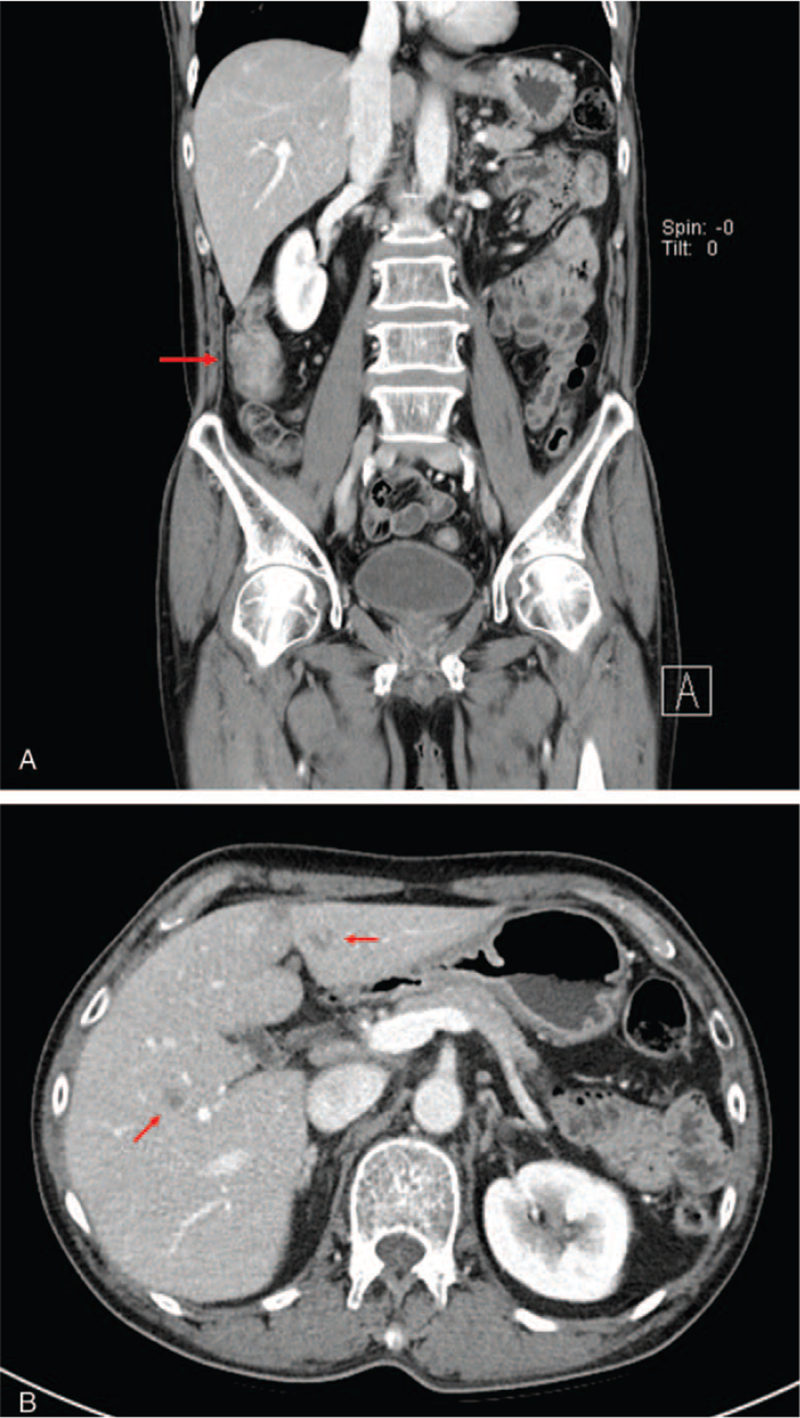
CT findings. (A) 4.3 cm size colonic mass in ascending colon (red arrow). (B) Ill-defined hypodense nodules in liver S3 (1.0 cm) and S5 (1.3 cm) (red arrows). CT = computed tomography.
